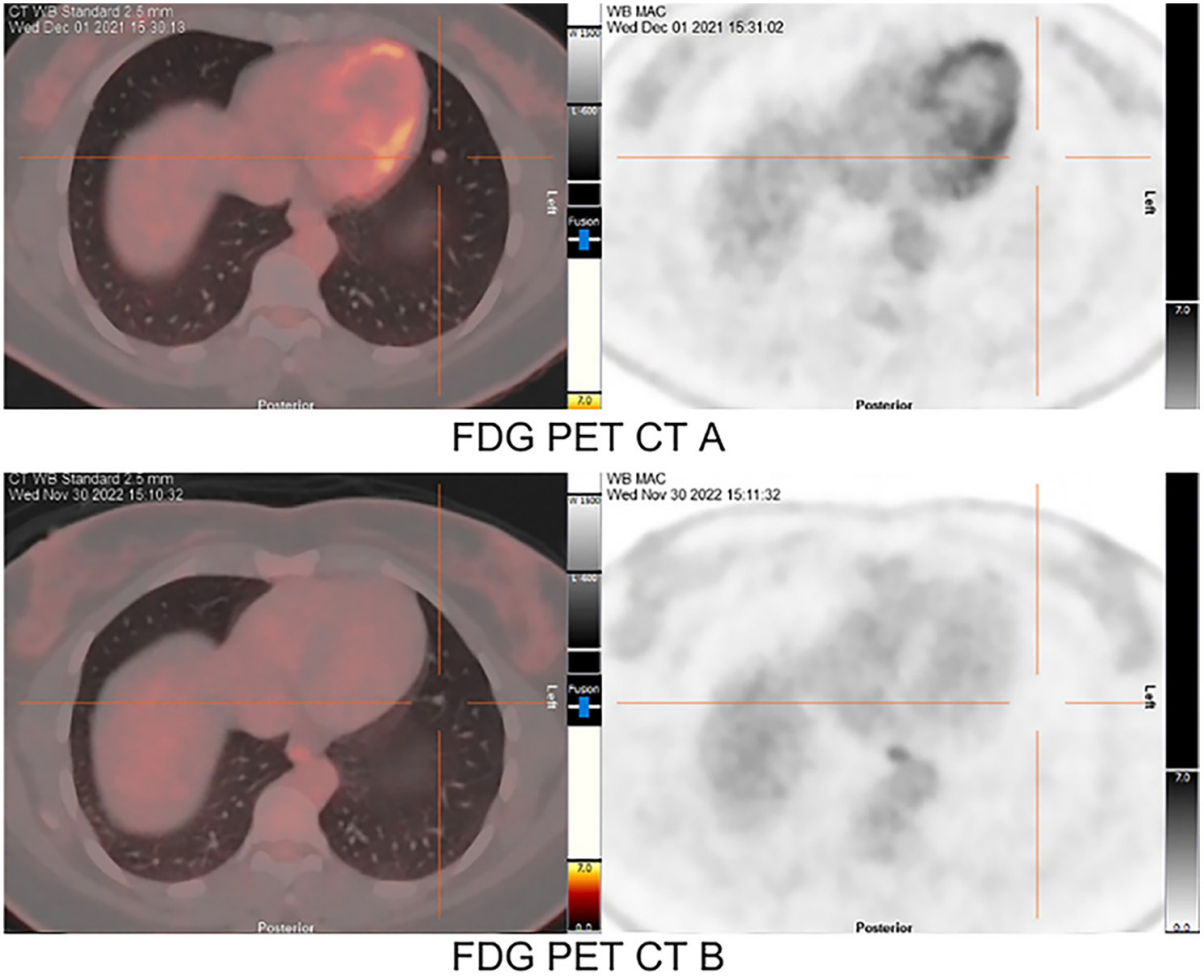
Case 2 FDG-PETs and maximum intensity projection (MIP) (right panels) obtained before and after larotrectinib treatment for target lesion 2. Pre-larotrectinib therapy FDG PET CT and MIP of the chest conducted on December 2021 in FDG-PET A. In comparison, FDG-PET B was obtained post larotrectinib treatment, indicating a reduction in size and decreased FDG-PET avidity of the target lesion, in November 2022.
